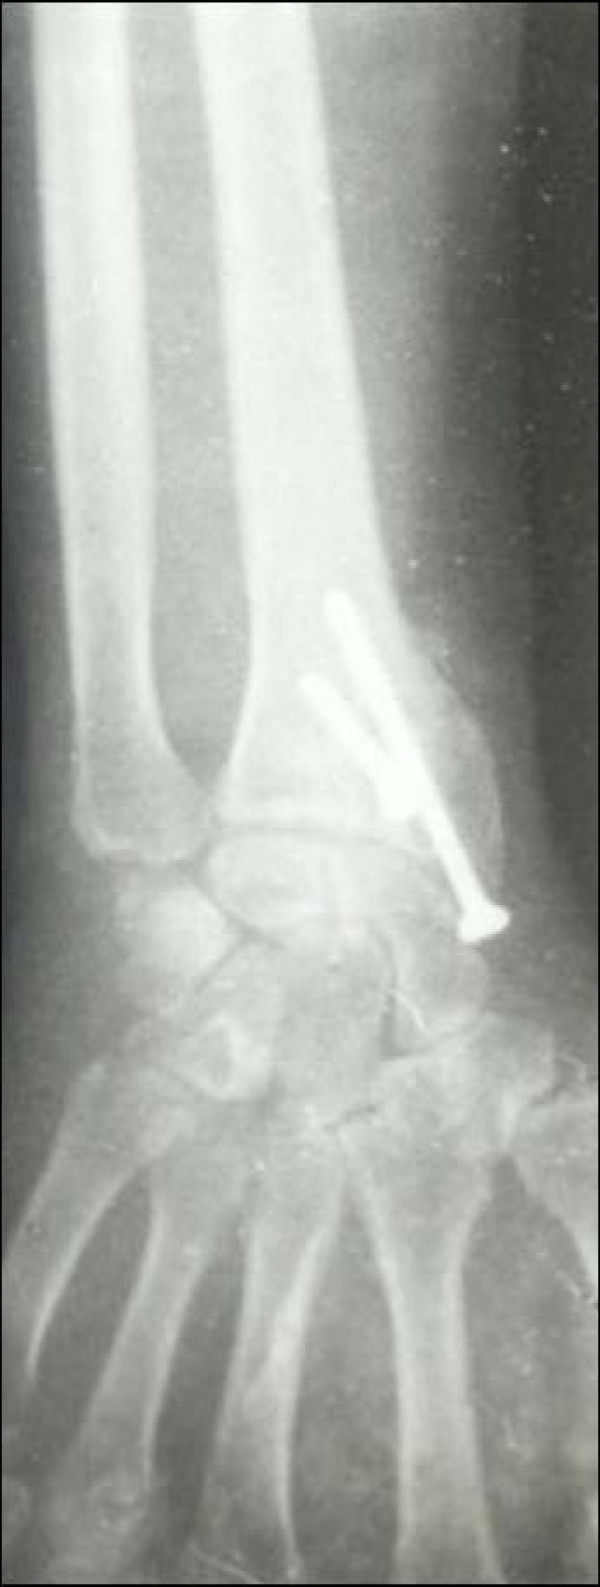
Case 1 Radiograph (Anteroposterior view) at 7 year followup.
